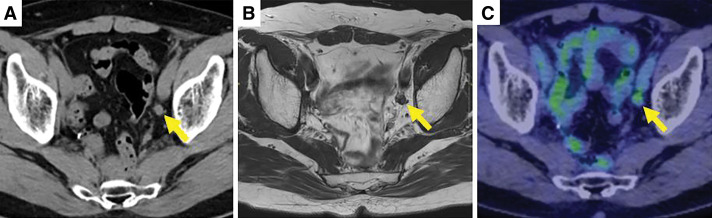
Plain CT, MRI T2 weighted, and PET-CT images taken 4.5 years after the initial surgery. CT and MRI show a 9-mm enlarged lymph node (arrows) (A, B), and PET-CT shows accumulation of SUVmax 2.08 (arrow) (C).
